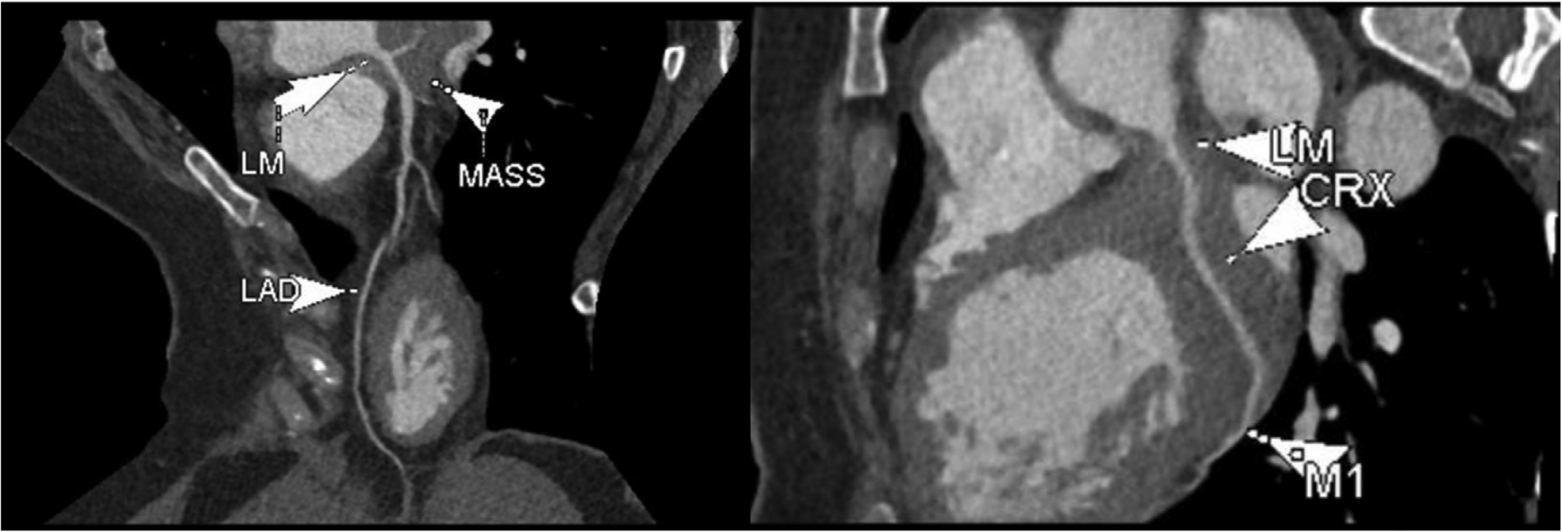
Cardiac Computed Tomography With Oblique Multiplanar Reconstruction The image shows the tumor encasing the left main (LM) and proximal left anterior descending (LAD) coronary arteries. CRX = left circumflex artery; M1 = first obtuse marginal artery.
